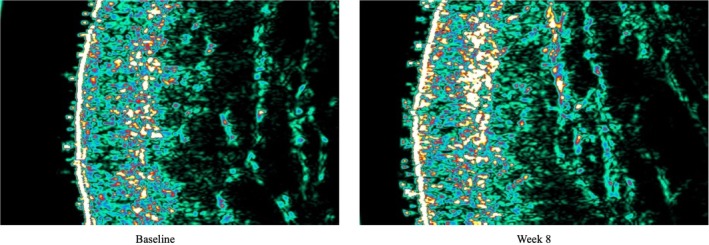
Ultrasound evaluation of dermal collagen density. The dermal collagen density was evaluated after 8 weeks of daily A.G.E. interrupter serum application and 1 session of fractional picosecond laser in a 67‐year‐old woman (case 7).
